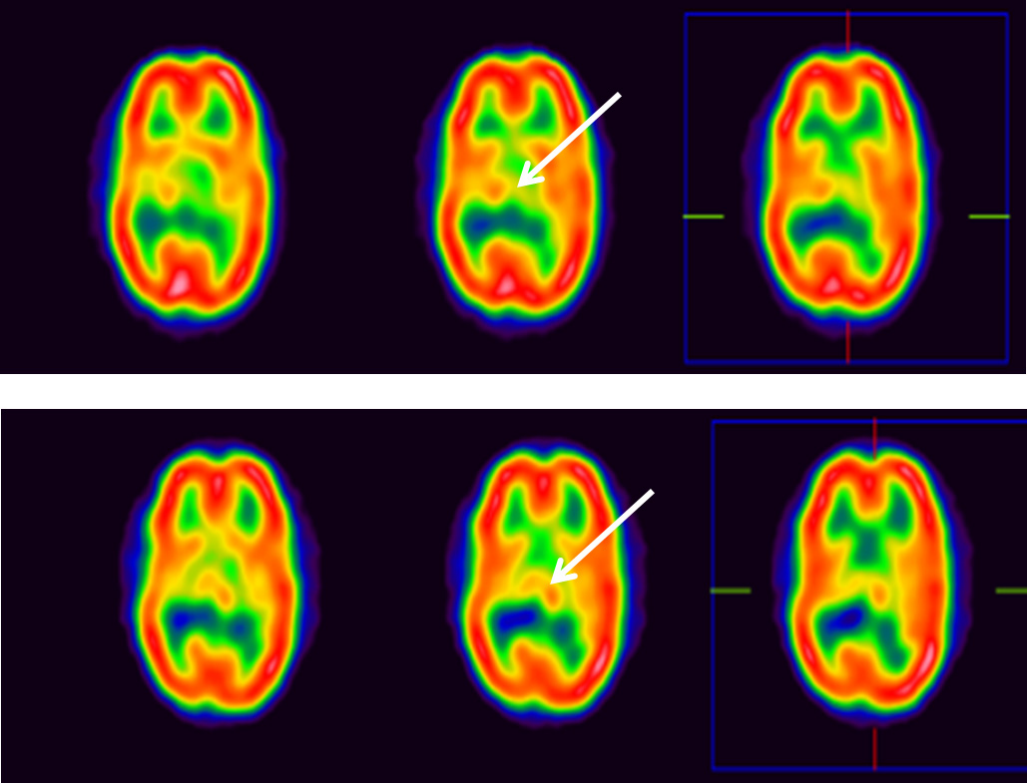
Brain perfusion SPECT analysis. The low perfusion in the left thalamus in the baseline study (white arrow, upper lane), has been corrected in subsequent images at 24 weeks (white arrow, lower lane) after infusion of cord blood.
